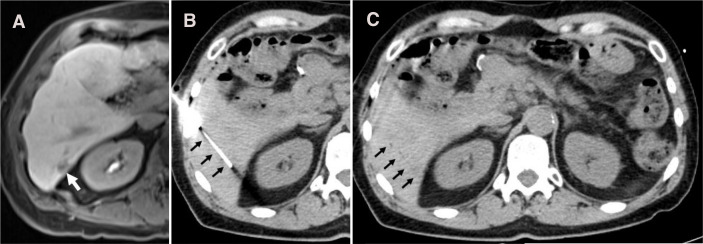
Case #5, Panel (A) Liver mass seen by delayed phase T1 post-contrast MRI (white arrow). Panel (B) Radiopaque needle seen during biopsy under CT guidance (black arrows). Panel (C) Thin line of gas (black arrows) seen on CT following electrocautery with the SinglePass device.
